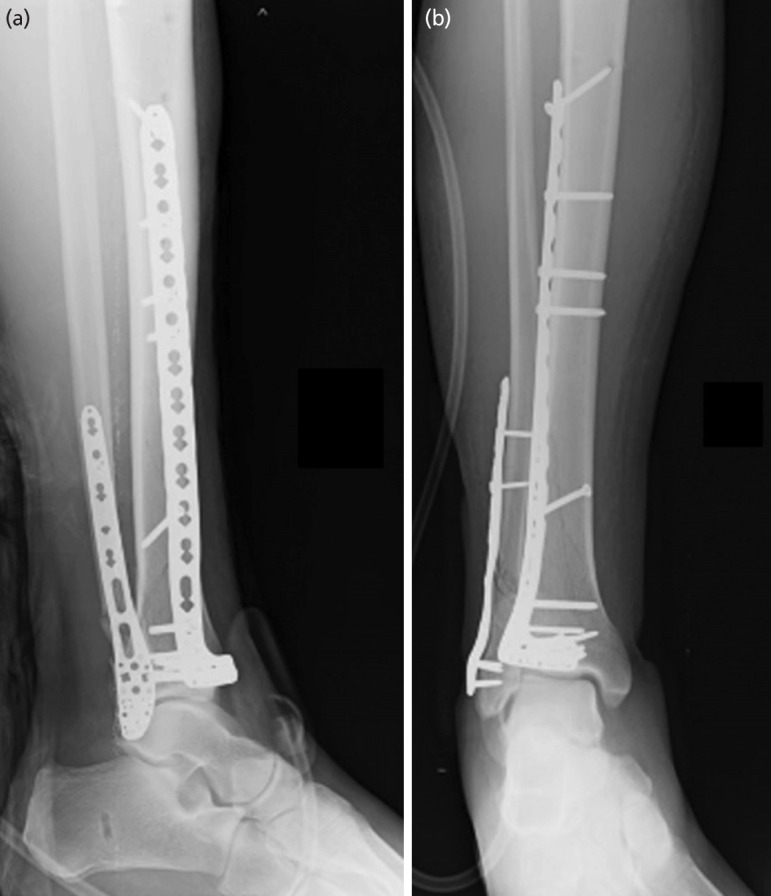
Post-operative radiographs. (a) Lateral view. (b) Antero-posterior view.
